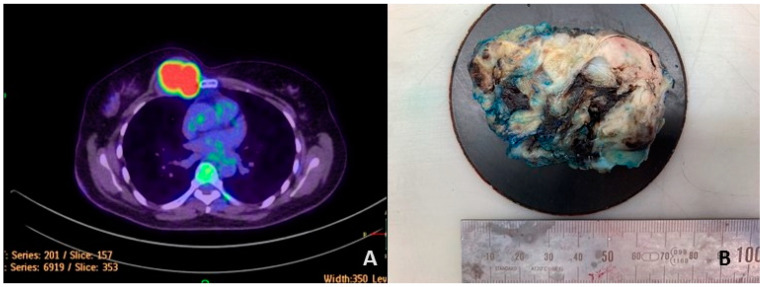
A positron emission tomography-computed tomography (PET-CT) image of the patient showed a right parasternal hypermetabolic rate tumor with thoracic wall and coastal invasion (A). The tumor shows a fleshy and greyish-white cut surface with a hemorrhage focus (B).
